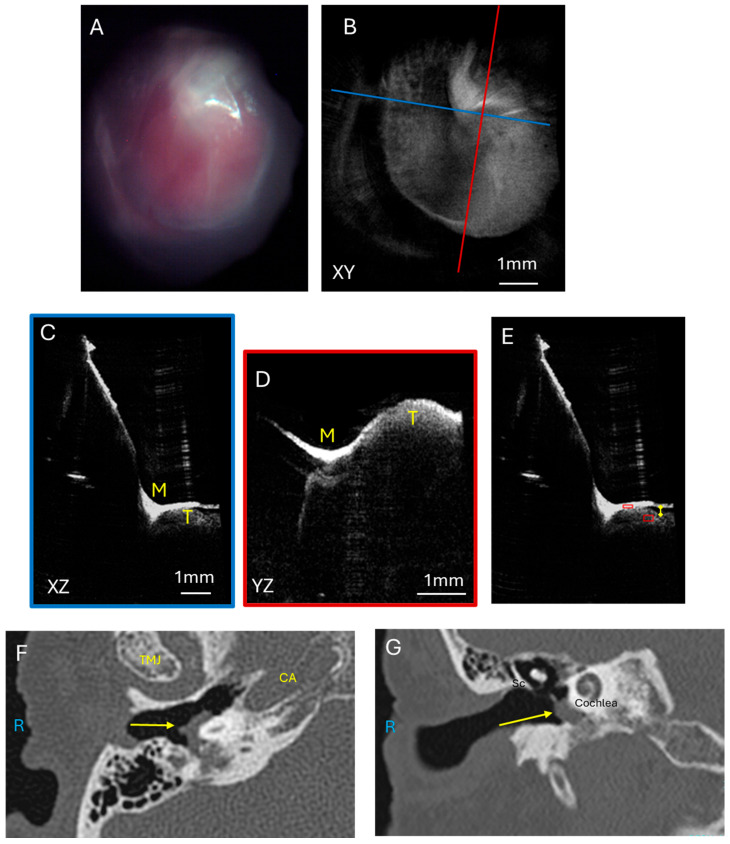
Glomus tumor. Endoscopic view of the right tympanic membrane (A) of a patient with a glomus jugulare with a large component of the tumor in the hypotympanum and mesotympanum. OCT enface XY view (B) of the tympanic membrane, with the blue line representing the plane leading to the middle ear for the cross-sectional XZ (C) view (blue box) and the red line representing the plane leading to the middle ear for the cross-sectional YZ (D) view (red box) showing the tumor which is touching the tympanic membrane and obstructing visualization of the middle ear ossicles. (E) Quantification of tumor pixel intensity in comparison to tympanic membrane pixel intensity (red boxes), and measurement of largest distance from the tumor to the TM (yellow line). Axial CT (F) showing the tumor (arrow) in the mesotympanic space, and coronal CT (G) showing the tumor (arrow) in the mesotympanic space. R, right side; M, malleus; Sc, scutum; T, tumor; TMJ, temporomandibular joint; CA, carotid artery.
